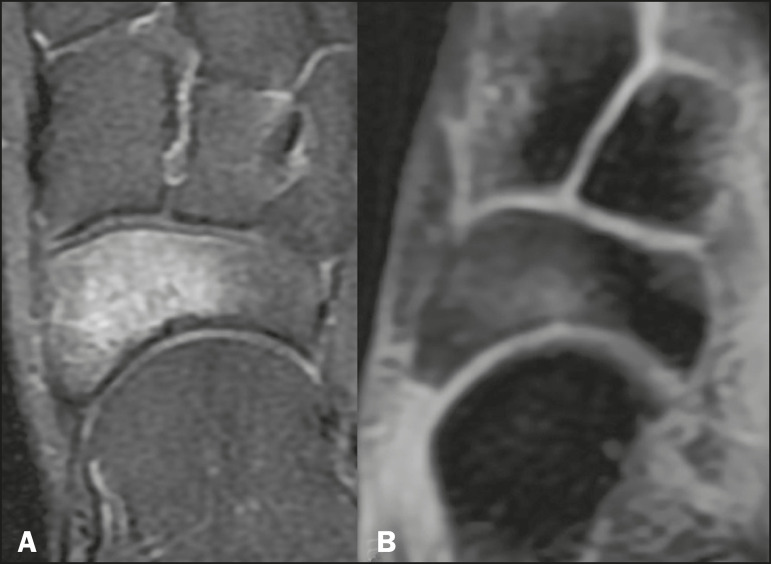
T2-weighted FSE sequence with fat suppression (A) and IDEAL water-only sequence (B) images of a 24-year-old female patient showing na osteochondral lesion in the navicular bone. The Dixon technique provides better delineation of chondral involvement, whereas bone marrow edema is more evident in the T2-weighted FSE sequence with fat suppression.
